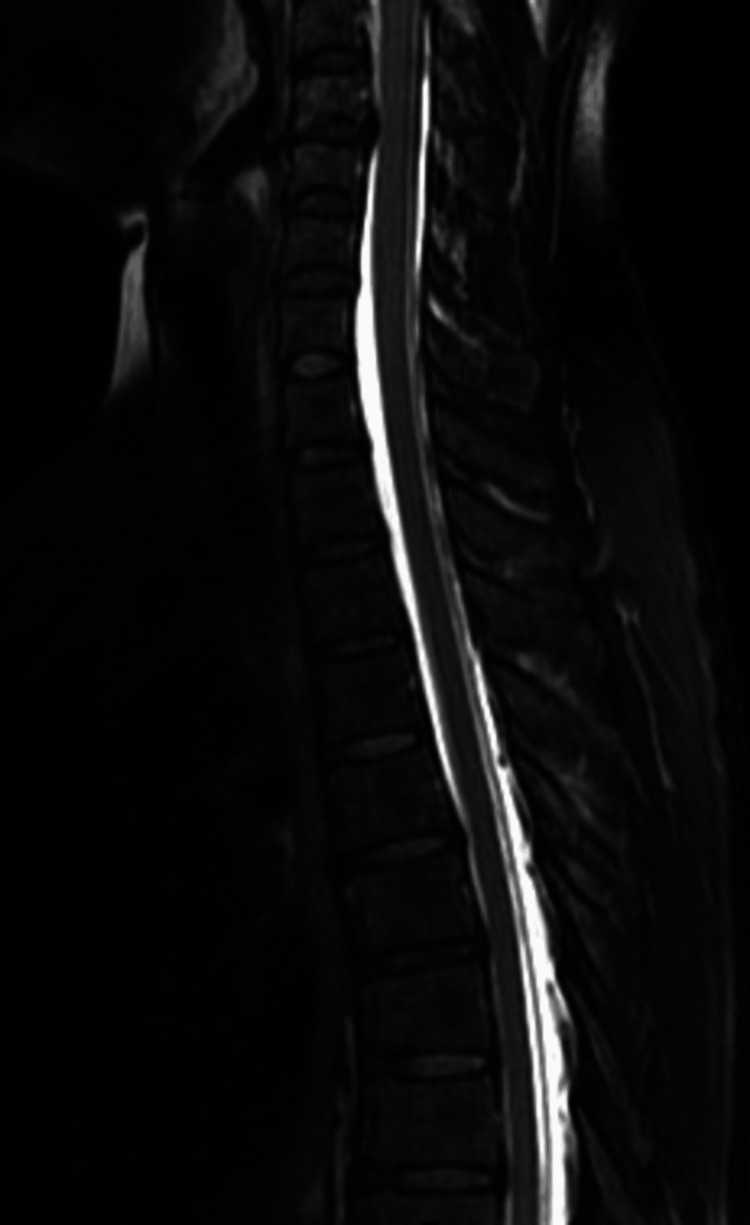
Magnetic resonance imaging acquired at admission. Sagittal T2-weighted imaging shows extensive cerebrospinal fluid collection in the extradural space of the third cervical vertebra to the sacrum. Water signal (white color) in the epidural space and the dural sac was noted.
